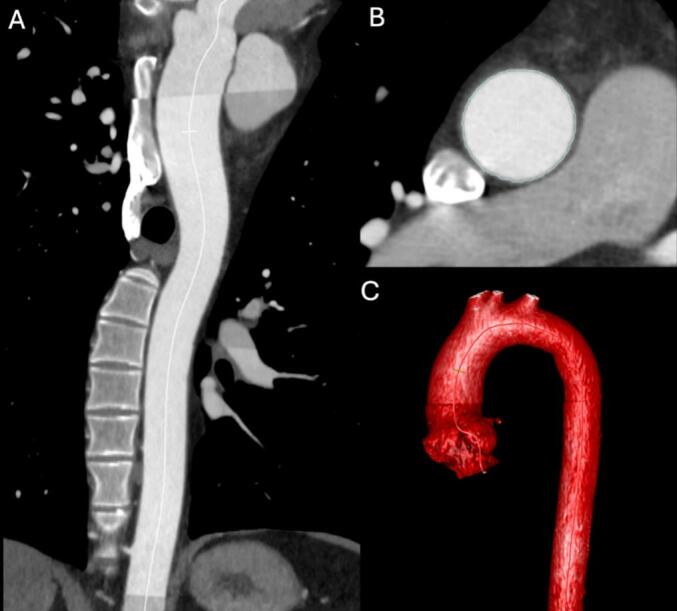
Post-processing of a thoracic aortic computed tomography angiography (CTA). (A, B) Centerline reformations of the thoracic aorta in mediastinal window settings, displaying orthogonal cross-sectional planes along the aortic lumen. Panel A shows the long-axis view, while Panel B shows the corresponding short-axis view perpendicular to the aortic centerline at the level of the ascending thoracic aorta. (C) Three-dimensional (3D) external volume-rendered (VR) reconstruction of the thoracic aorta.
